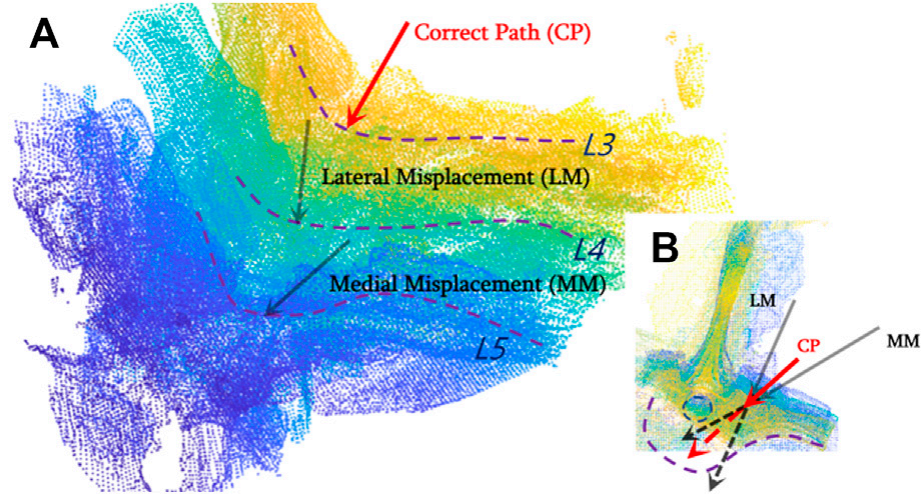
Drilling entry point determination and coarse path planning based on co-registered CT and US images for pedicle screw implantation. (A) Three types of screw implantation paths (correct path, lateral misplacement, and medial misplacement) are defined on co-registered CT and US images; (B) 2D visualization of three typical screw implantation paths.
